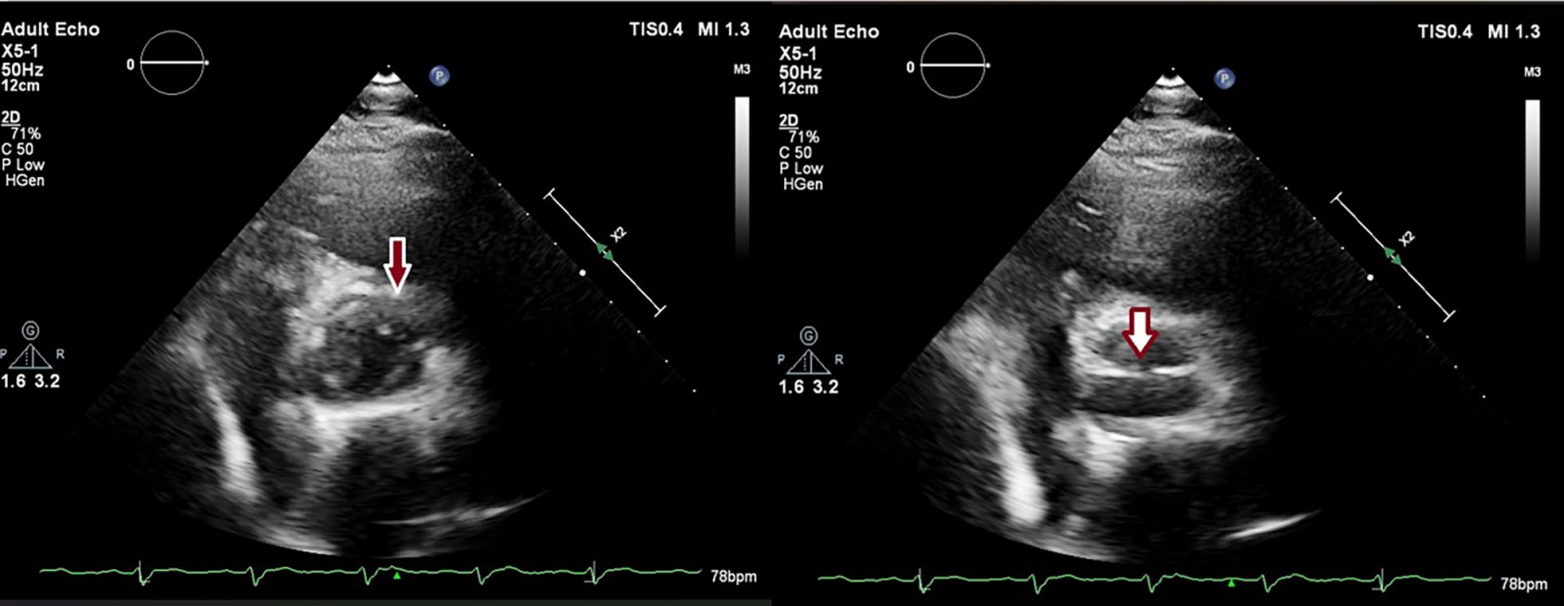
Transthoracic echocardiogram (TTE) image in parasternal short axis showing aortic valve in systole (red solid arrow) and in diastole (white solid arrow). Note the single commissural line in diastole
